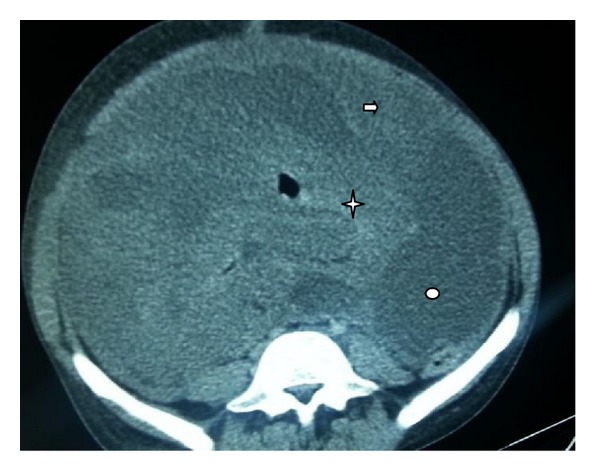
Computed tomography scan of abdomen showing an important thickening of small bowel (star), with ascites (oval) and nodules of peritoneal carcinomatosis (arrow).
